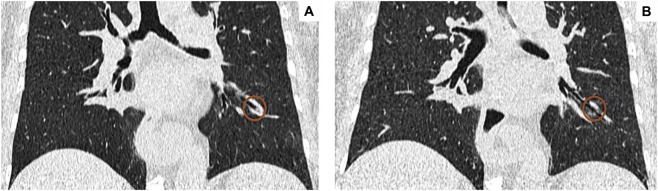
Effects of tezepelumab on mucus plugs as assessed by chest high-resolution computed tomography (HRCT) before (A) and after (B) 12 months of treatment.
